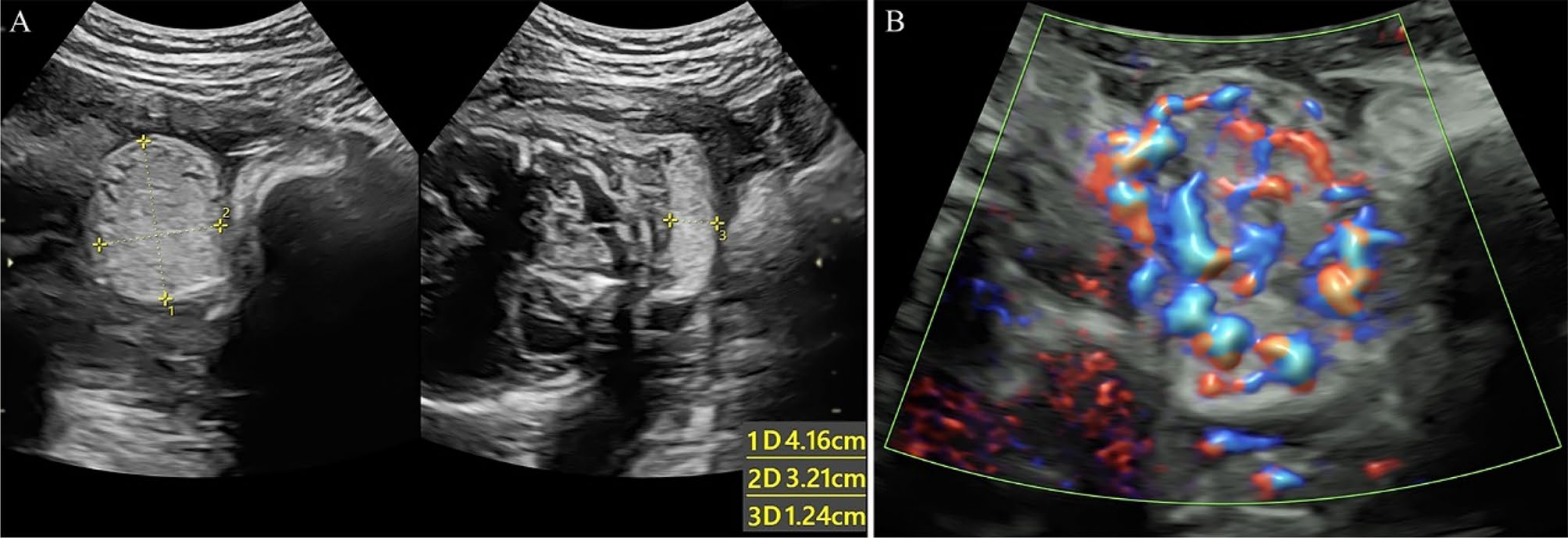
A hemangioma at 26 weeks of gestational age. (A) Prenatal ultrasound exhibited a hyperechogenic lesion (4.16 × 3.21 × 1.24 cm) located at the left of the neck. (B) Color Doppler ultrasound of blood flow showed rich blood flow signals inside the mass.
